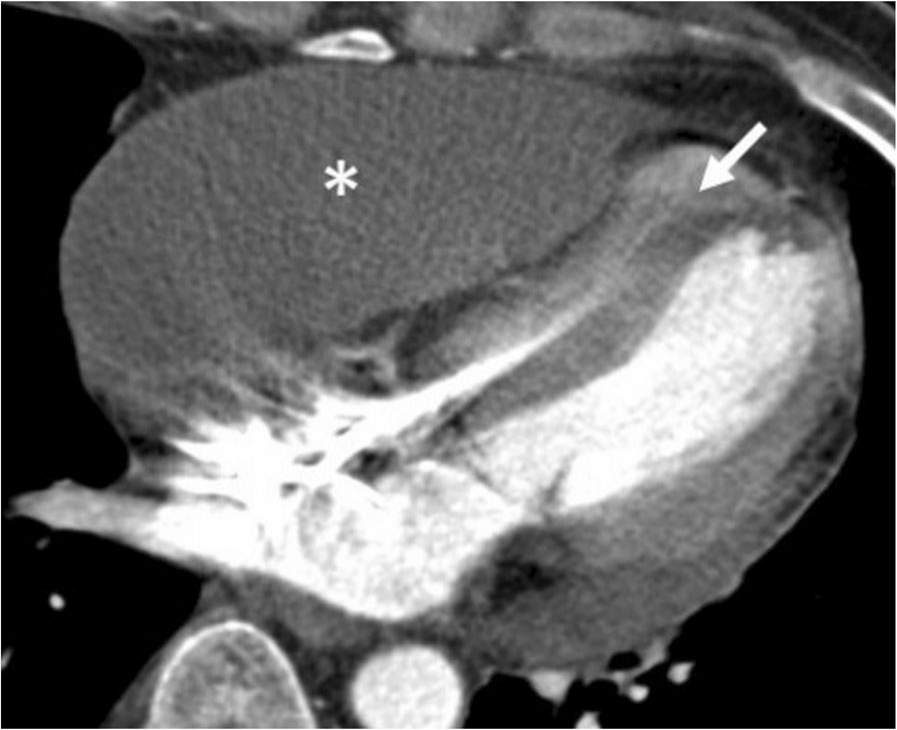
A 61-year-old man with right heart failure symptoms underwent to CT scan to rule out the presence of pulmonary artery embolism. Axial contrast-enhanced CT image shows significantly compressed right ventricle (arrow) due to the loculated pericardial effusion (asterisk)
